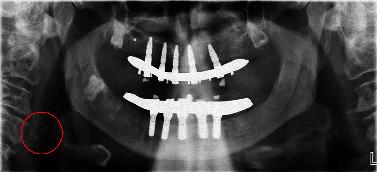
Typical location of atheroma plaque in orthopantomography. (Own source). In the image, we can observe a calcified lesion in the carotid region in front of C3-C4 following the anatomy of the artery.
